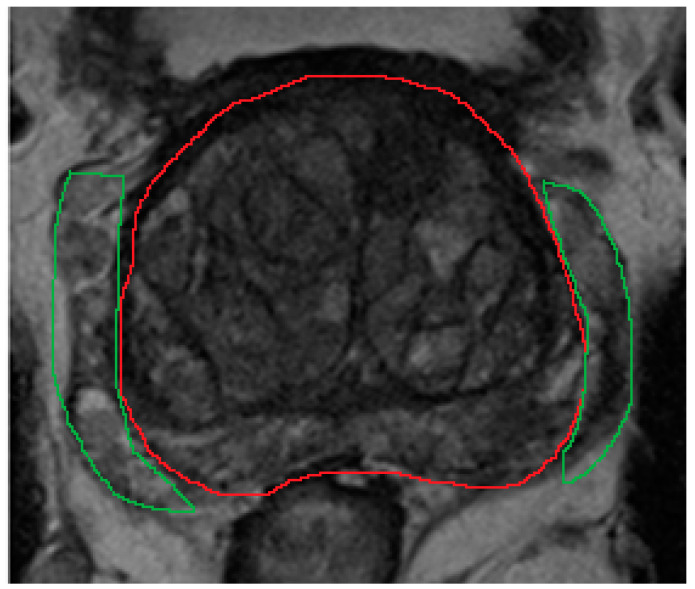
On MRI, the periprostatic venous plexus appears as serpinginous hyperintense structures with foci of signal voids adjacent to the prostate (green outline), and can be closely related to the prostate capsule (red outline). It may have similar heterogeneous appearance as the peripheral zone. Therefore, during manual segmentation, it can be mistaken as part of the prostate to less experienced operators.
